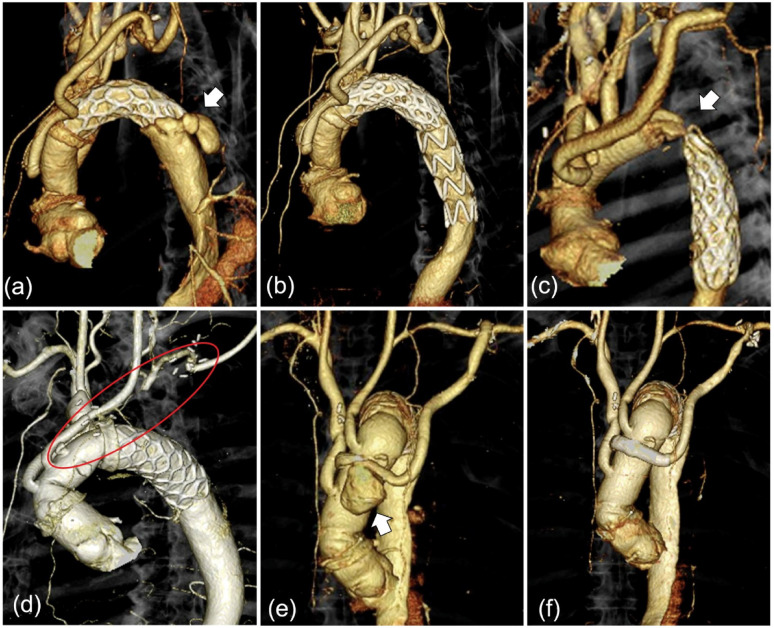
CT findings of aorta- and LSA bypass-related events. (a) Distal stent graft-induced new entry (arrow) and (b) its exclusion after TEVAR. (c) Stenosis at the non-stented frozen elephant trunk segment (arrow), which was subsequently treated with TEVAR. (d) LSA bypass graft occlusion (circle). (e) Pseudoaneurysm at the LSA anastomosis site (arrow) and (f) its exclusion with a peripheral stent graft. CT, computed tomography; LSA, left subclavian artery; TEVAR, thoracic endovascular aortic repair
